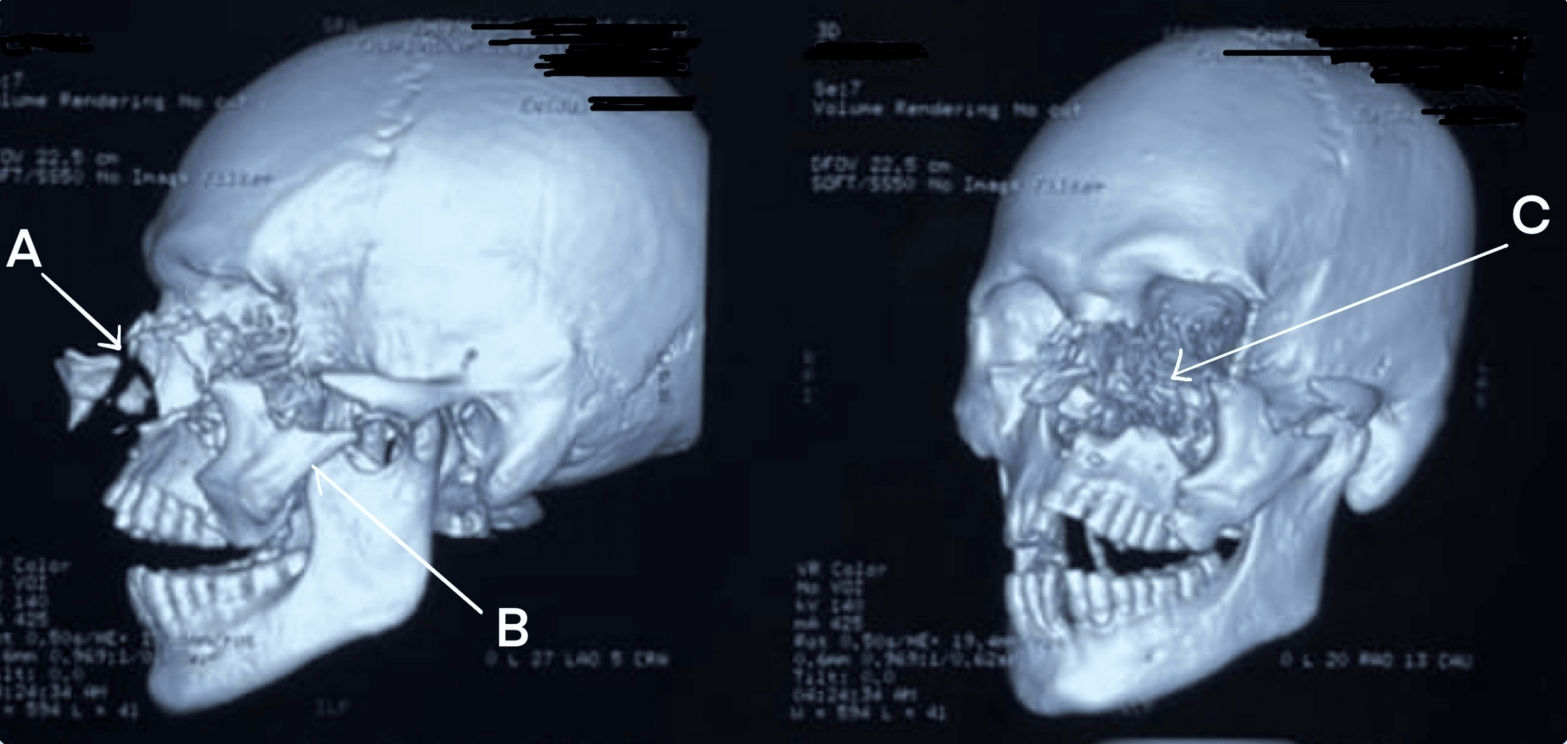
3D CT of the face and skull (Case 3) (A) Fracture of the left nasal bone. (B) Fracture of the left zygomatic process. (C) Comminuted fracture of the medial wall of the left orbit.
